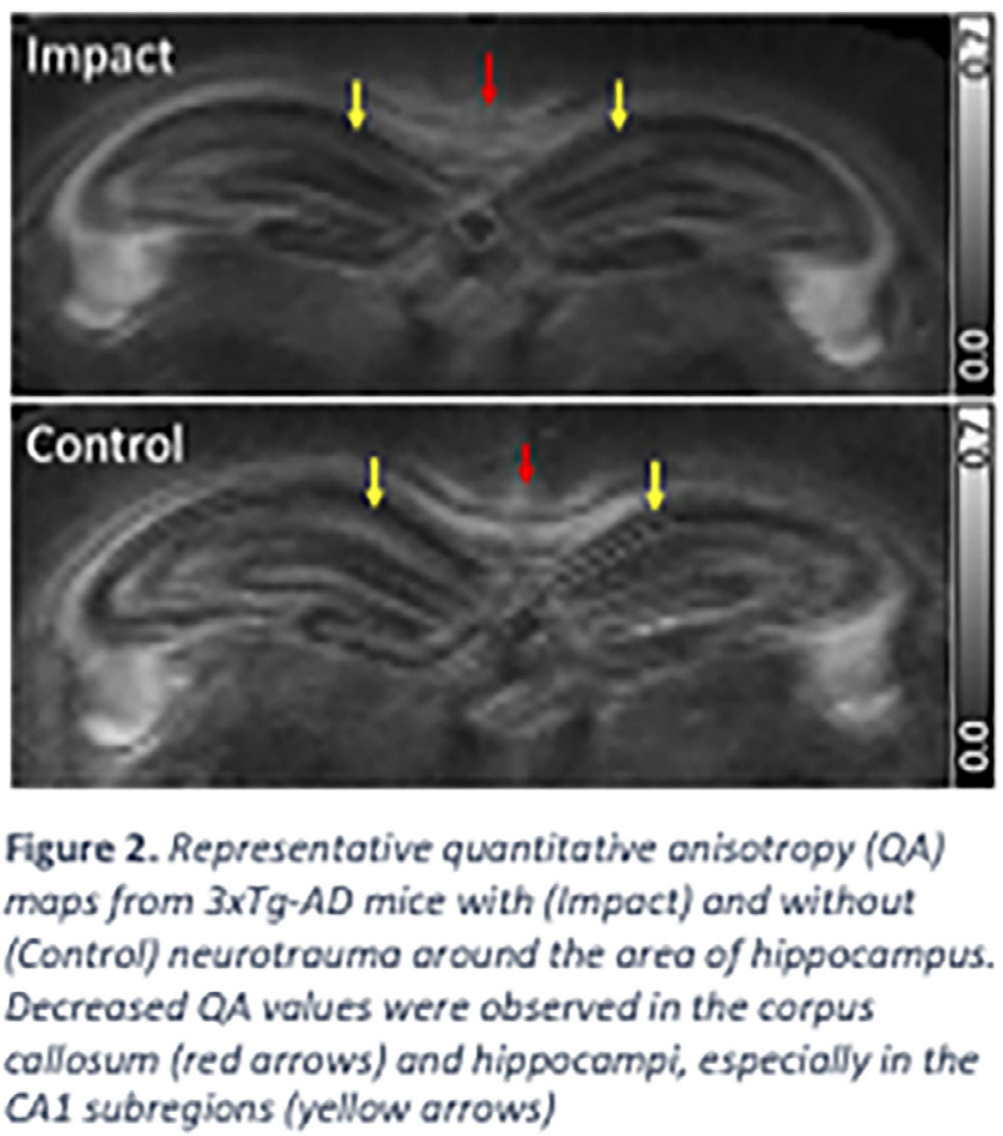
# Application of High‐Resolution Diffusion‐MRI in Neurotrauma Accelerated Alzheimer's Disease
